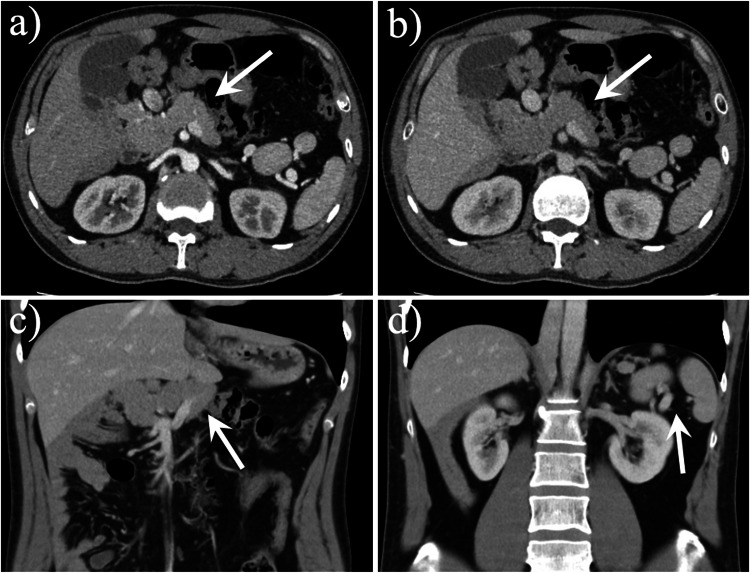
A 32-year-old man with dorsal pancreatic agenesis associated with polysplenia syndrome. Axial contrast-enhanced computed tomography (CT) images (a, b) and coronal contrast-enhanced CT image (c) showed the pancreatic head (arrow) and neck and the absence of part of the pancreatic body-tail portion. Coronal contrast-enhanced CT image (d) showed multiple accessory spleens in the left upper quadrant (arrow)
